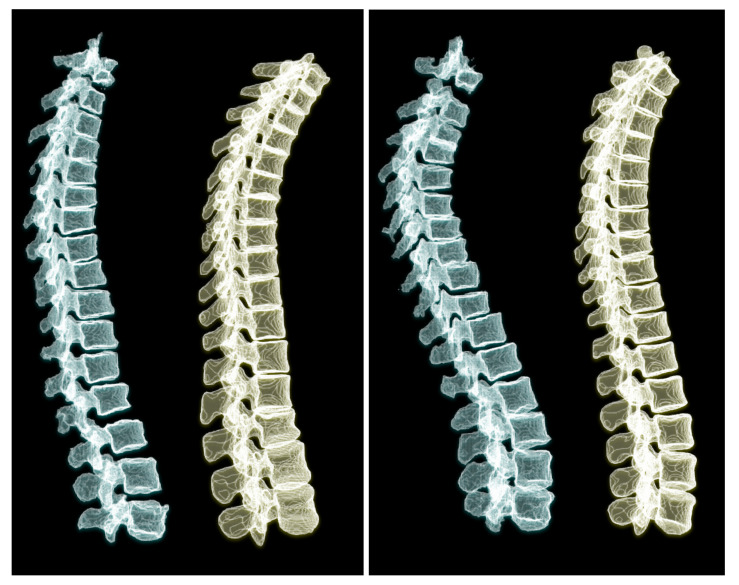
Comparing 3D reconstructions of the standing spinal posture from clinical radiographs (left, green) to the spinal posture of the same patient in lying-down position from CT imaging (right, yellow) in two different patients. In the upright-standing posture the spine is under natural weight bearing, which leads to a different spine curvature.
